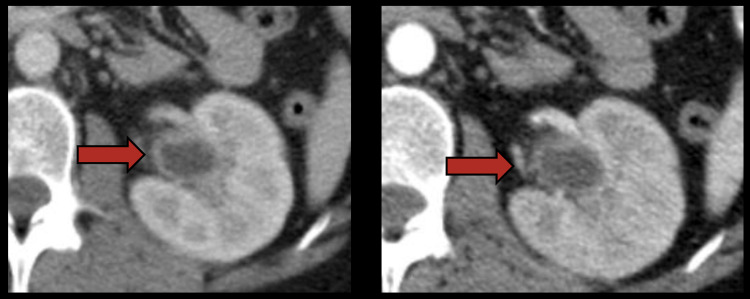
CT showing thickening of the renal pelvis (4 mm) with a slight urothelial dilation (right kidney).
